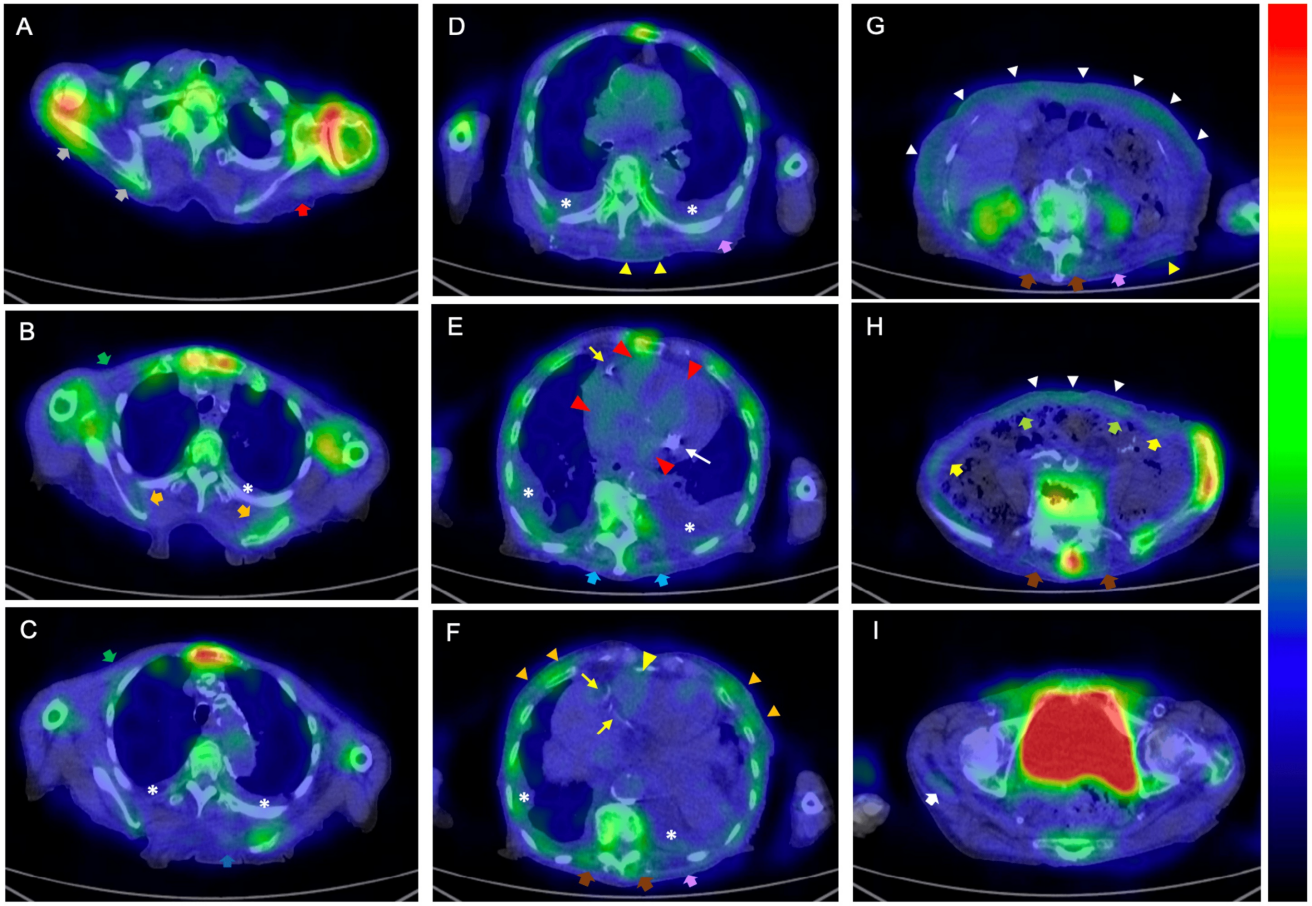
SPECT images of technetium-99m-labeled pyrophosphate (Tc-99m-PYP) scintigram with fusion to CT images obtained 2 hours after injection of the radiotracer Horizontal plane of the (A) shoulder, (B–D) upper chest, (E, F) heart, (G, H) abdomen, and (I) pelvis. In SPECT/CT fusion images displayed in color, Tc-99m-PYP uptake at the sternum is considered the maximum, whereas that at other sites is expressed as relative values. Tc-99m-PYP uptake in the region of interest is considered positive when shown in a color indicating approximately 40% or more of the uptake in the sternum (yellow–green on the color bar) and negative when shown in a color indicating less than 40% (blue). There is no tracer uptake in either the atrial or ventricular myocardium. The red arrowheads indicate cardiac pool tracer uptake. Accumulation of Tc-99m-PYP in the deltoid (red arrow), infraspinatus (gray arrows), subscapularis (orange arrows), pectoralis major (green arrows), rhomboid (blue arrow), latissimus dorsi (purple arrows), trapezius (light blue arrows), erector spinae (brown arrows), abdominal oblique (yellow arrows), rectus abdominis (light green arrows), and gluteus (white arrow) muscles, mammary gland (orange arrowheads), subcutaneous back (yellow arrowheads), and subcutaneous abdominal fat (white arrowheads) is shown. Stars, thin yellow arrows, and thin white arrows indicate pleural effusion, calcification of the coronary arteries, and mitral annular calcification, respectively. SPECT: Single photon emission computed tomography
